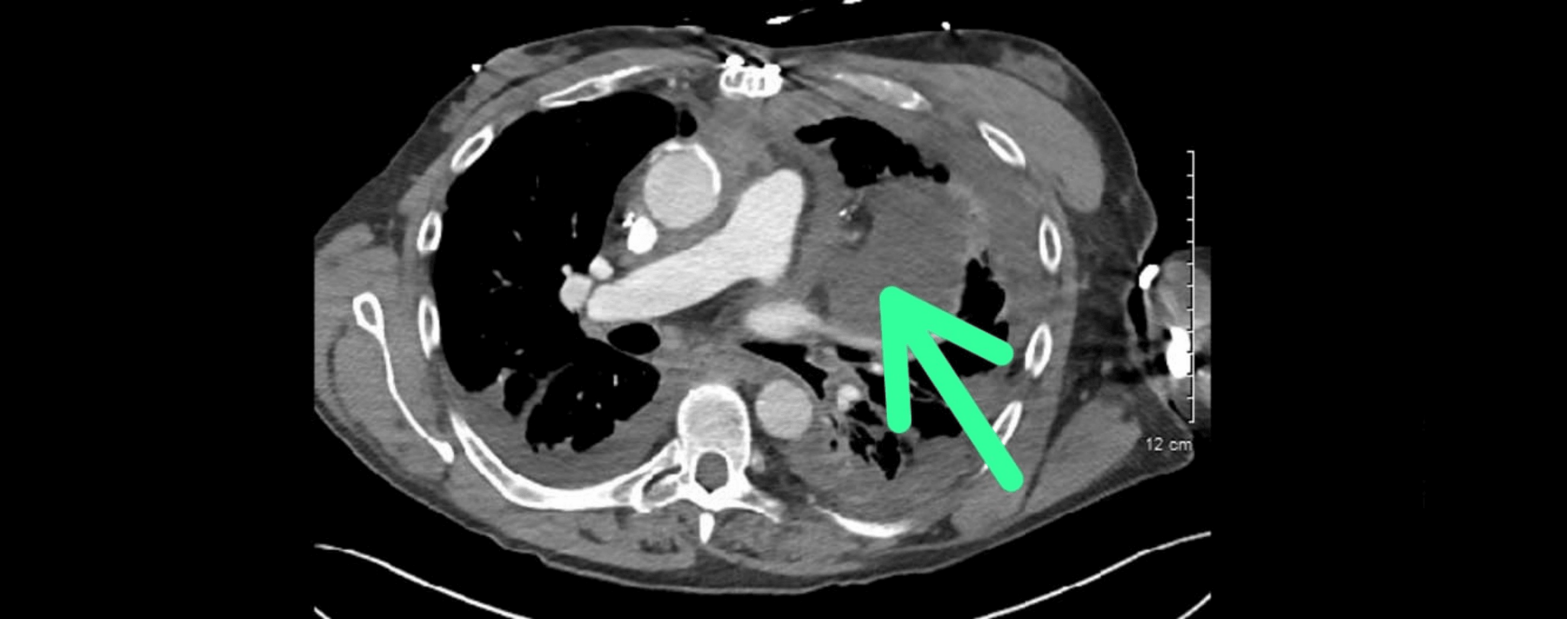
Cross-sectional view of CT angiogram of the chest showing opacities originating from the left pericardial fat (green arrow) CT: computed tomography
